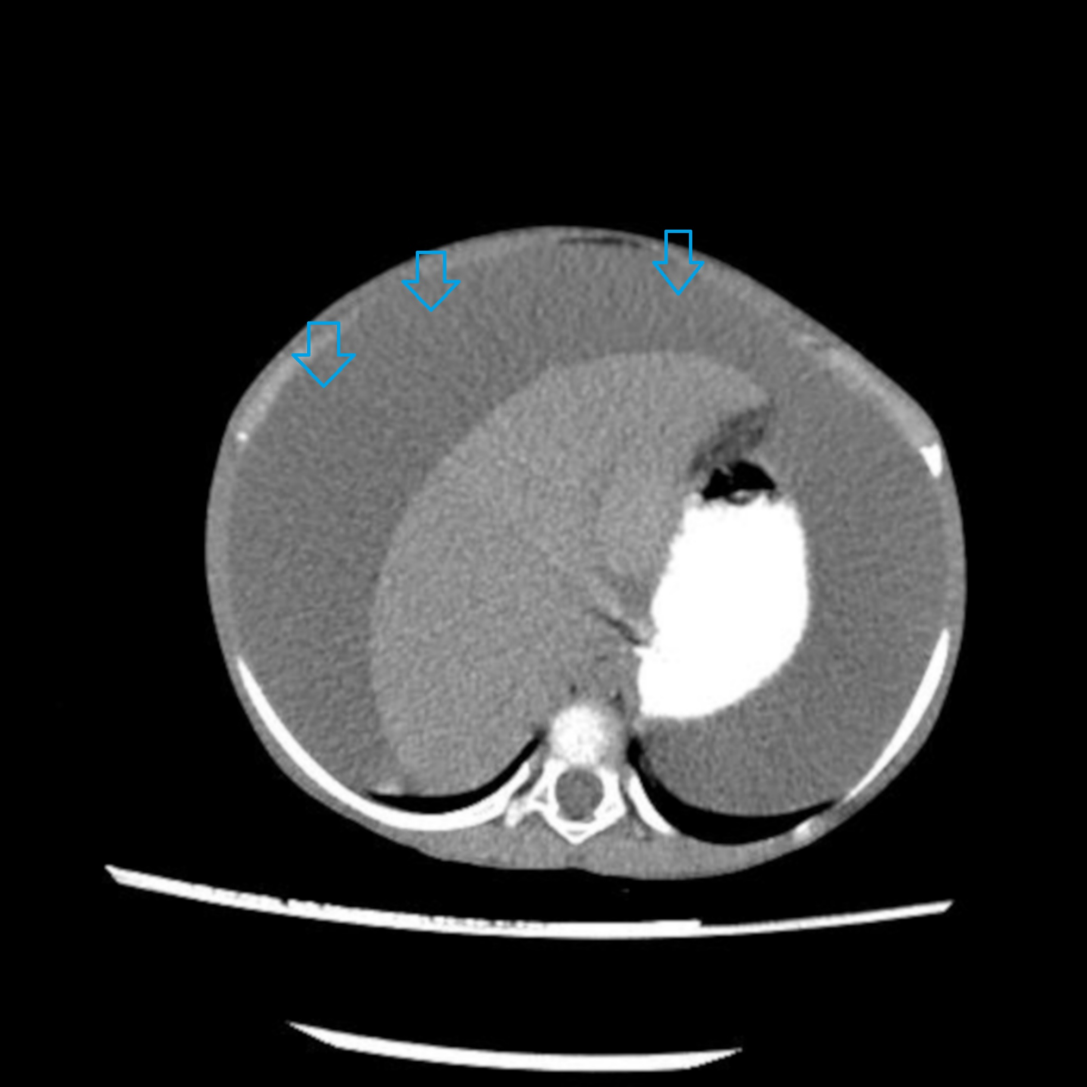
Computed tomography (CT) scan of abdomen with contrast showed gross ascites (arrows).
